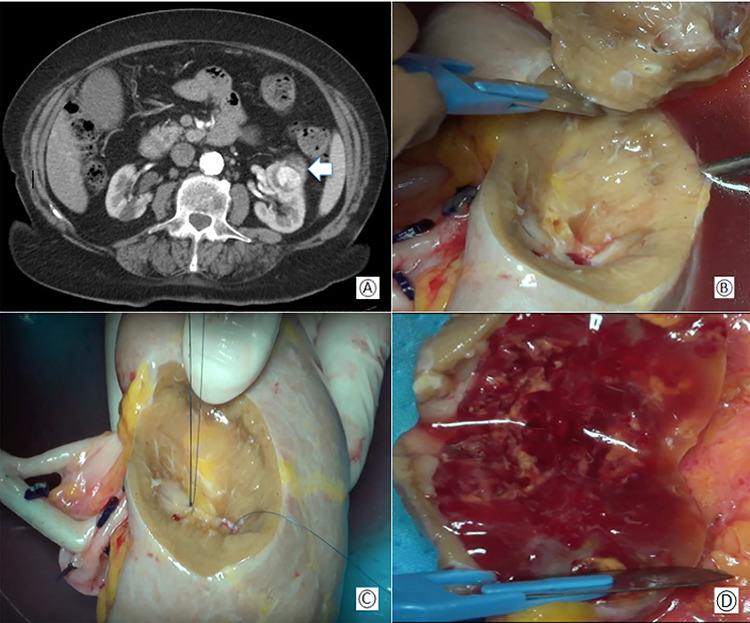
(A) Preoperative computed tomography: the white arrow shows the renal mass; (B) removal of the tumor during bench surgery; (C) suture of the renal calices during bench surgery; (D) macroscopic examination of the surgical piece.
